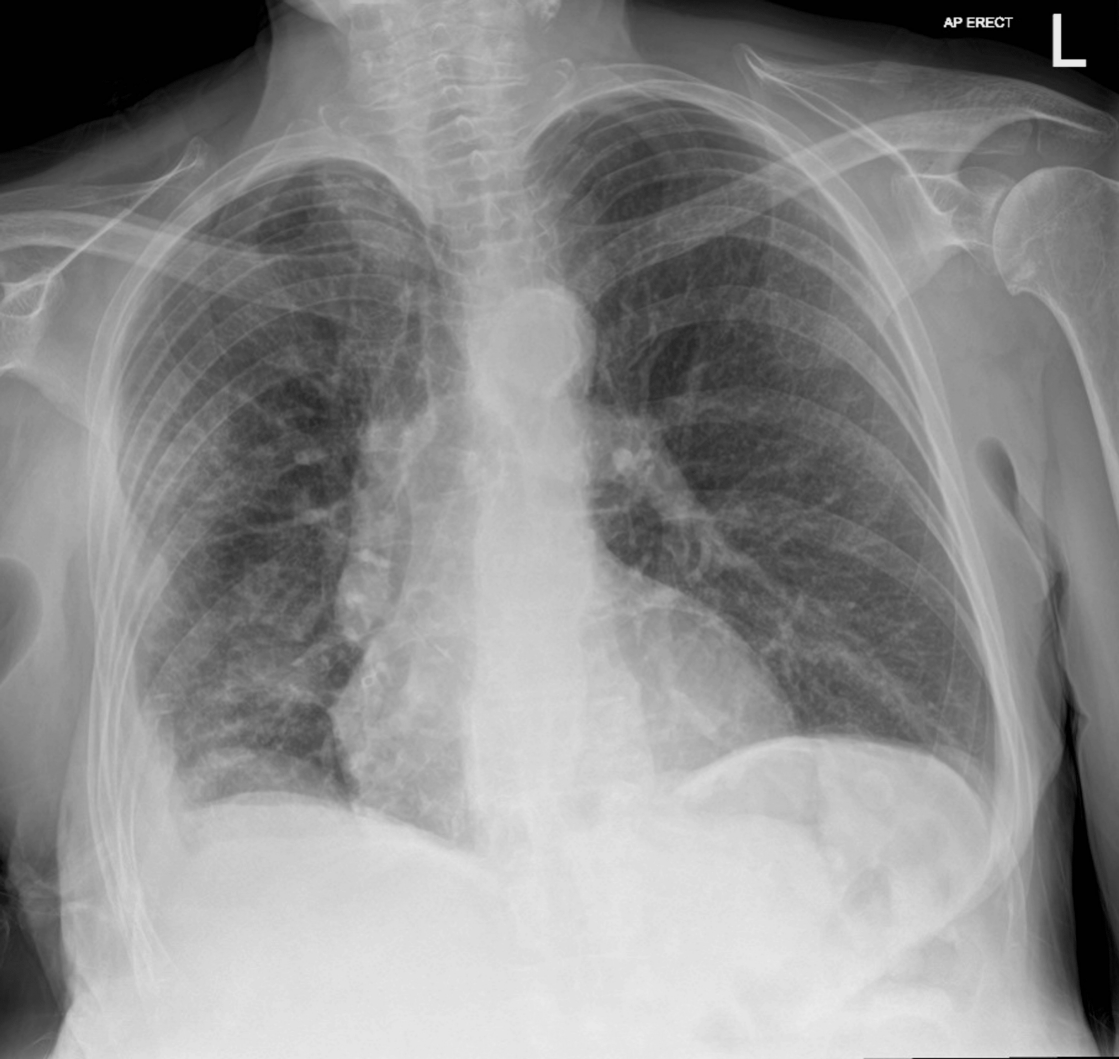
Chest X-ray two months after discharge
